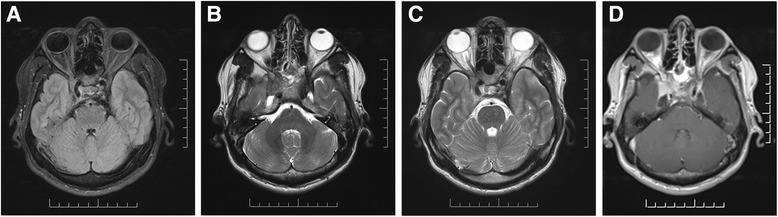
Axial T1 (a) and T2 (b, c) weighted magnetic resonance imaging, pre-gadolinium, showing an osteodestructive skull base process, with a lesion centered around the *right* sphenoid sinus and extending through the cavernous sinus into the adjacent meninges and *right* superior orbital fissure. Axial (d) T1 weighted magnetic resonance imaging, post-gadolinium, showing the focus of enhancement of the lesion
